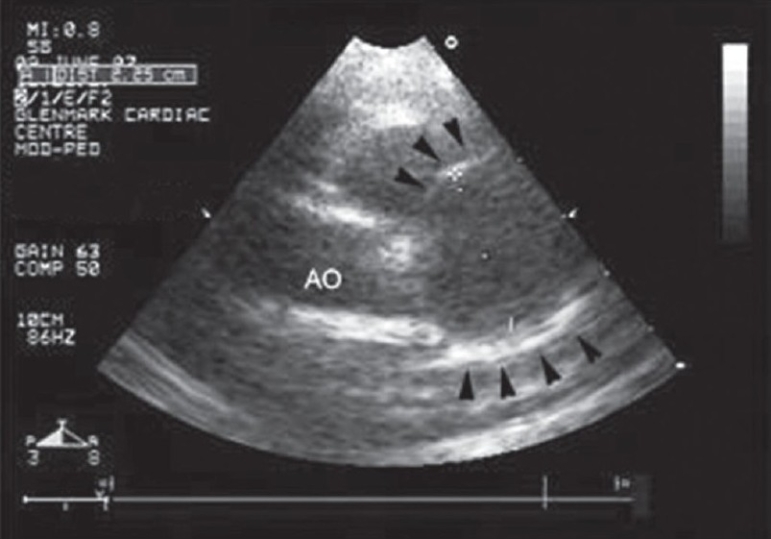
Follow-up echocardiography in short-axis view at the level of aortic valve demonstrating increase in the size of aneurysm (arrow heads) with complete resolution of the thrombus
